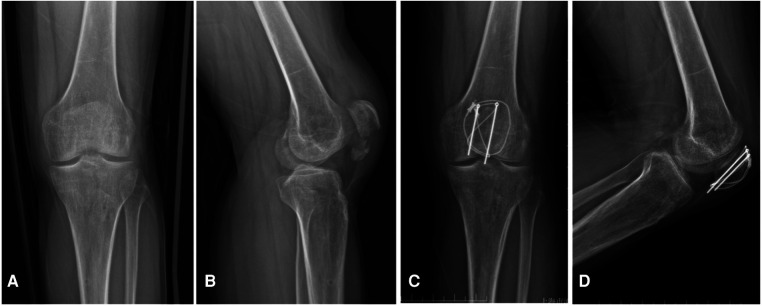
Case example of displaced inferior patellar pole fracture (Male, 36 years, left knee) using the ring-pin combined with cable cerclage. Preoperative radiographs in anteroposterior (A) and lateral (B) views showing a displaced inferior patellar pole fracture. Radiographs in anteroposterior (C) and lateral (D) views at 3 months follow-up, which show that the fracture was healed and the implants were in position without failure.
